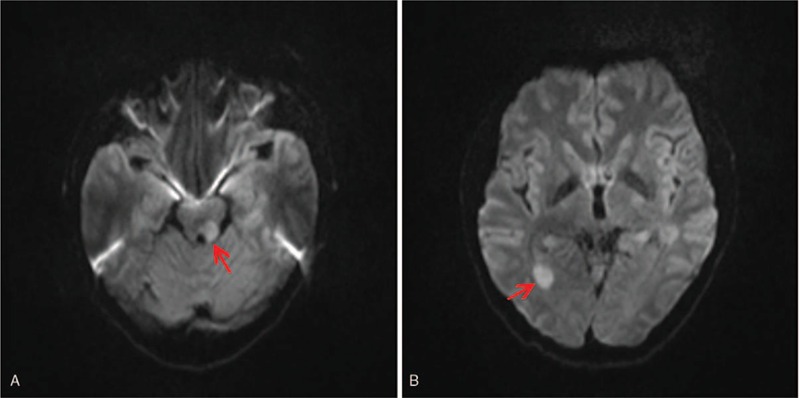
Brain diffusion-weighted magnetic resonance imaging (MRI-DWI) of the anti-IgLON5 disease. Representative axial, MRI-DWI showed asymmetrical areas of reduced diffusion involving left tegmentum of the midbrain (A; arrowhead) and right occipital horn of the lateral ventricle (B; arrowhead).
